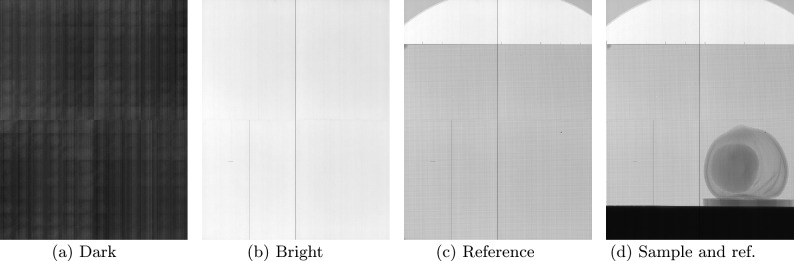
Experimental images required for the spatial harmonic imaging acquisition procedure, using a hazelnut as a test object. (a) Dark image: acquired with the X-ray source switched off, characterizing the detector’s intrinsic noise. (b) Bright image: captured with the X-ray source on and without sample or optical components, providing the flat-field illumination reference. (c) Reference image: recorded with the inverted Hartmann mask in place, essential for subsequent phase retrieval. (d) Sample image: acquired with the hazelnut positioned in the beam and the optical component maintained in the same position as in (c).
